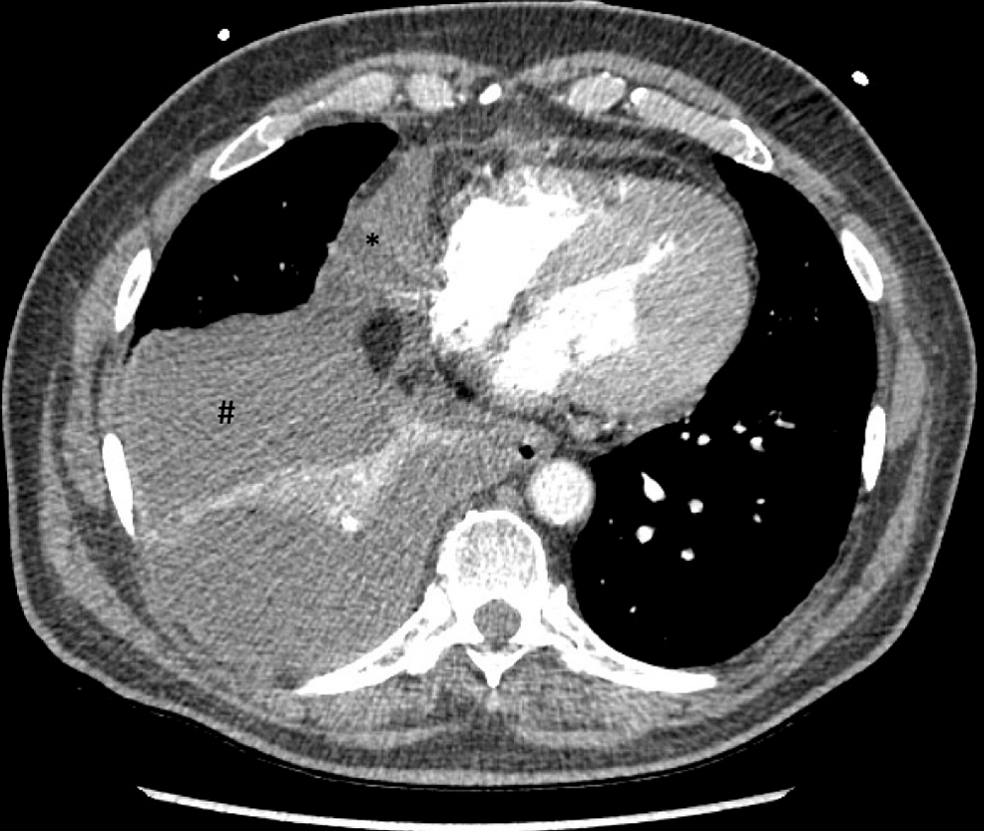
Transverse view of CT angiogram obtained on admission revealing a significant right pleural effusion (#) and right pericardial effusion (*).
